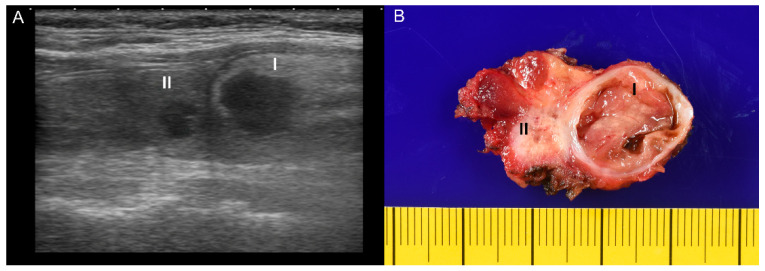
(A) The ultrasound examination of both thyroid nodules, referred to as the nodule I (2.3 × 1.9 × 1.4 cm) and the nodule II (1.0 × 1.0 × 0.6 cm), showed hypoechoic and heterogeneous. (B) The gross image of the right thyroid from thyroidectomy revealed one well-defined, encapsulated cystic nodule measuring 2.0 × 1.5 × 1.3 cm (I) and one ill-defined, white fibrous nodule measuring 0.7 × 0.6 × 0.6 cm (II).
